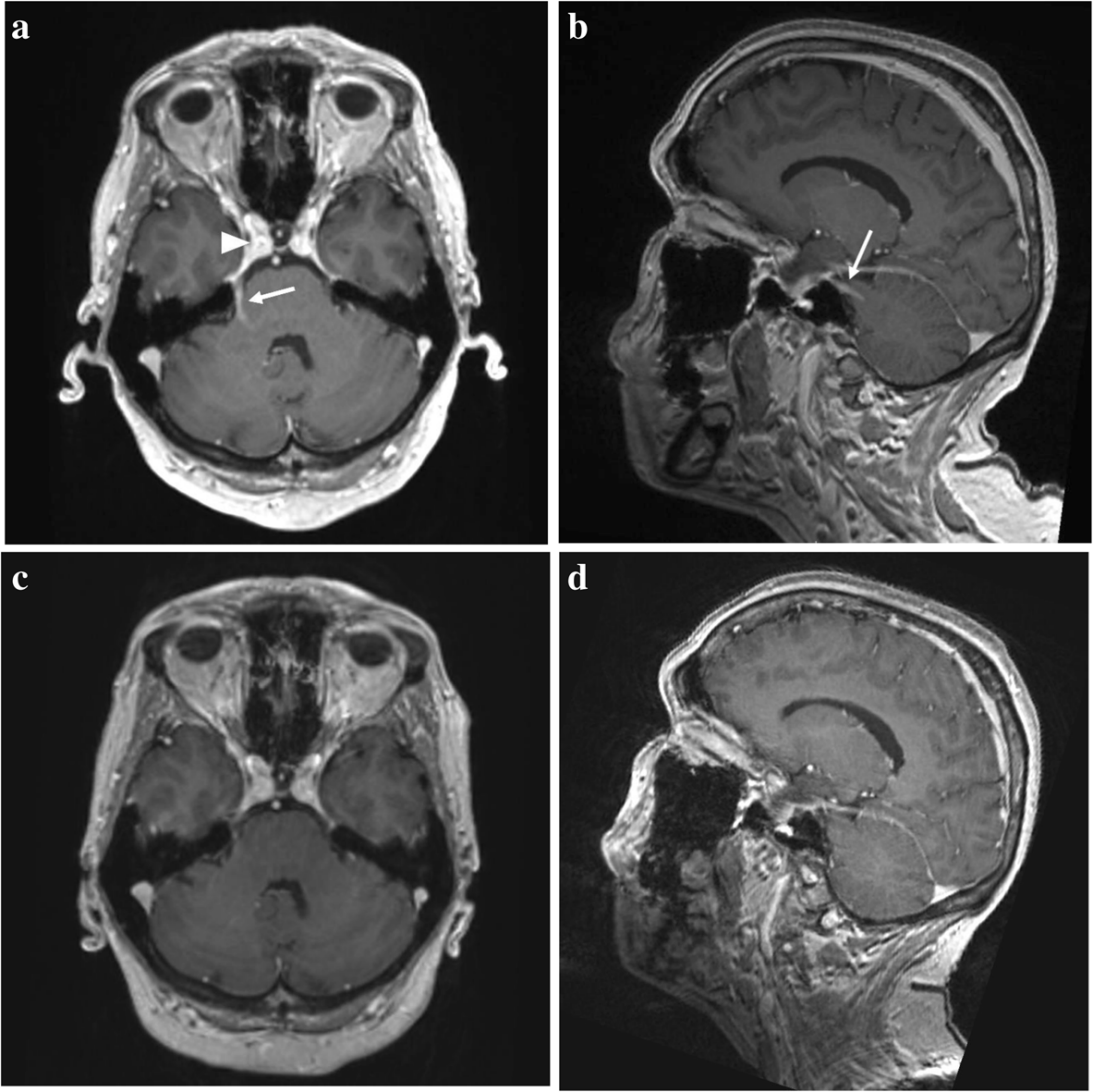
Brain MRI. Sagittal plane (a) and axial plane (b) showing enhancement of the trigeminal nerve (arrow) from the brainstem to the Gasser’s ganglia (arrowhead). This enhancement disappeared during the treatment [sagittal plane, (c), and axial plane, (d)]
